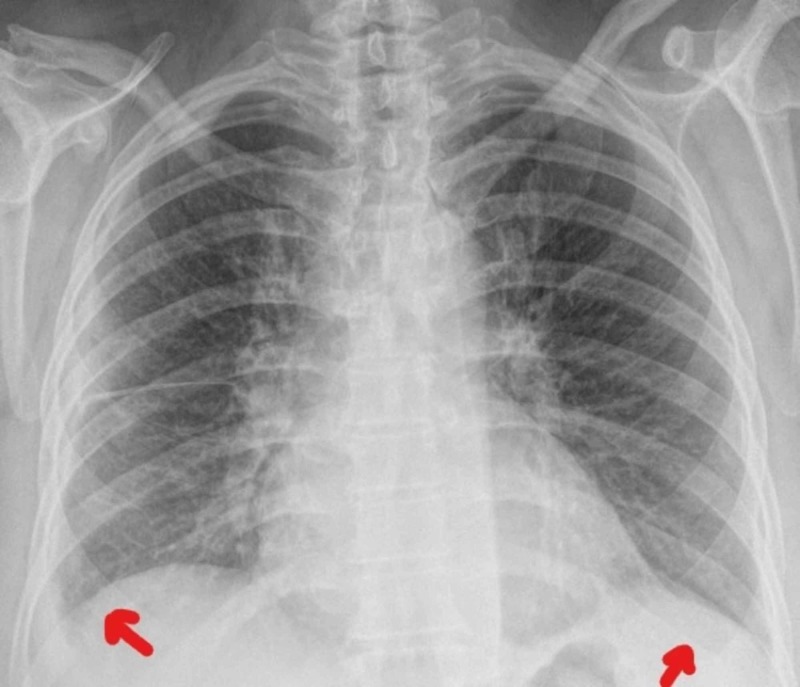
Thoracic radiography from day 19
